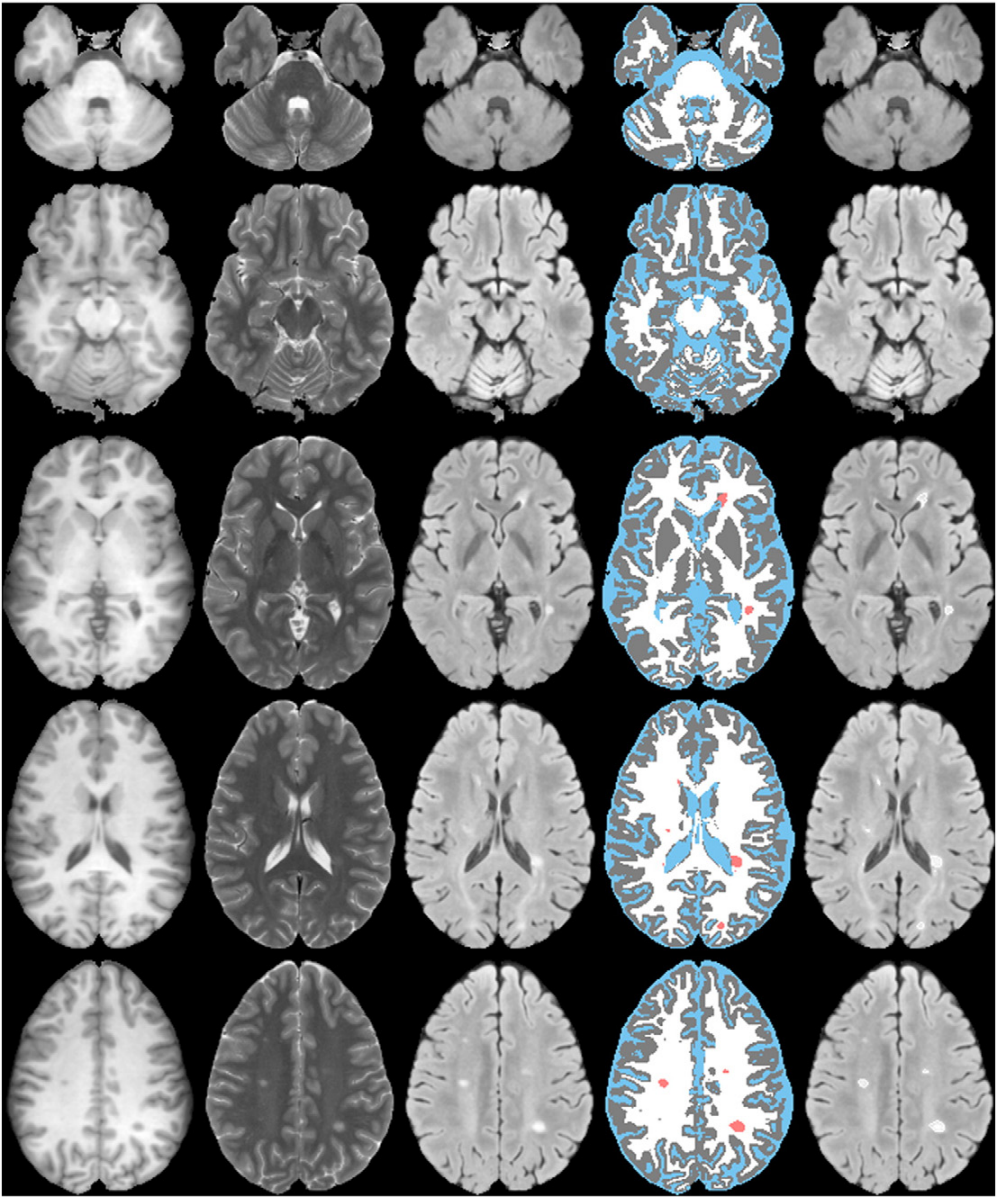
Automated segmentation at various cross-sections of the MICCAI private data CHB_test1_Case13. First column: T1; second column: T2; third column: FLAIR; fourth column: segmented; and fifth column: boundaries of the segmented lesions superimposed on FLAIR images. Color scheme is same as Fig. 2.
